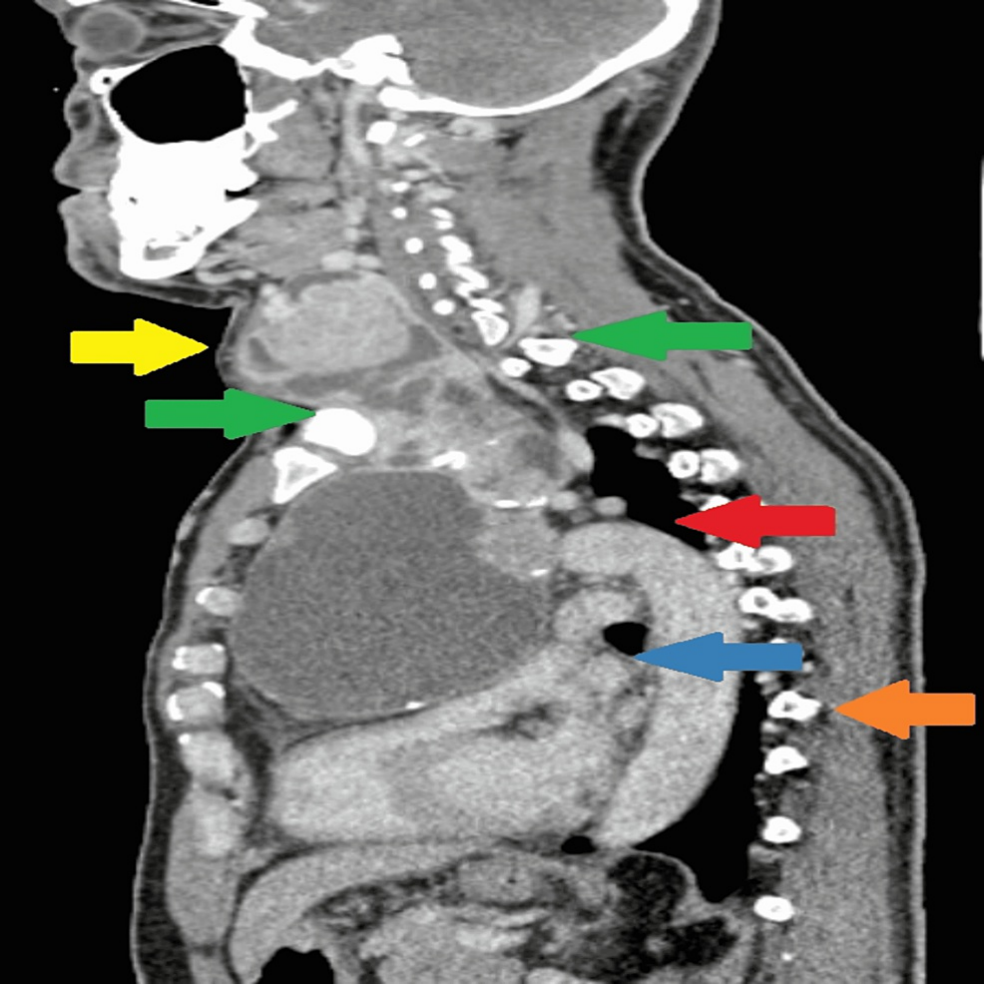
CT (sagittal plane) of the goiter extending below thoracic inlet into mediastinum. Yellow arrow indicates solid cystic component of goiter at the cervical region, green arrow indicates goiter extending below thoracic inlet, upper border of the manubrium (front) to upper border body of first thoracic (behind), red arrow indicates arch of the aorta, with the goiter extending below the arch of the aorta, blue arrow indicates the compressed superior vena cava, and orange arrow indicates goiter’s inferior border extending till T9 vertebra.
